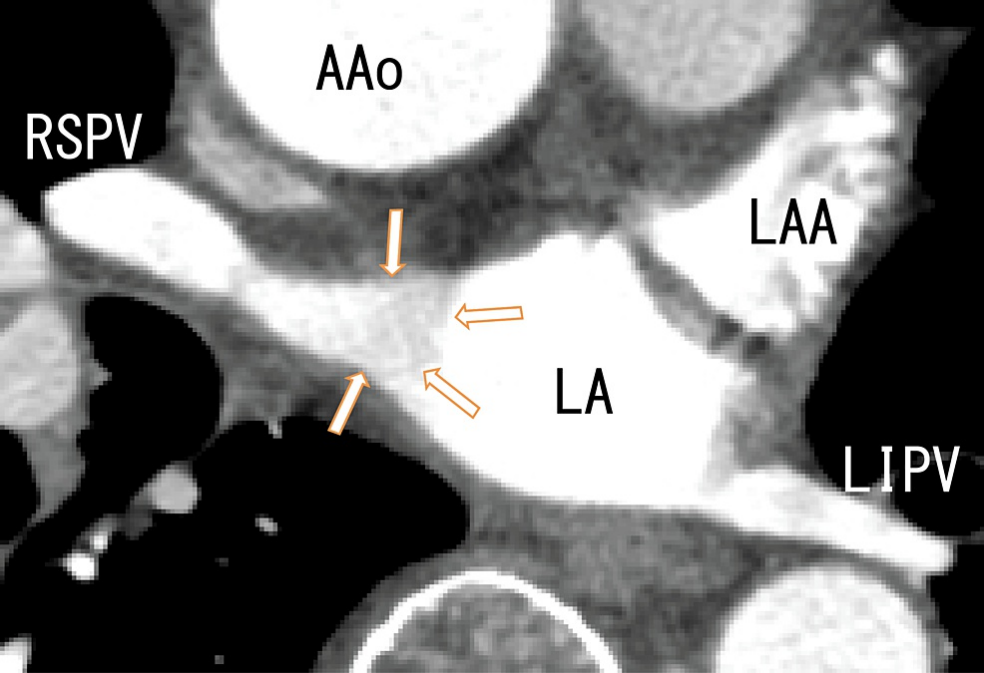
Axial images from an 80-MDCT scan obtained after five months of standard-dose warfarin remedy showing LA thrombi Axial images from an 80-MDCT scan obtained after five months of standard-dose warfarin remedy showing RSPV, LIPV and LA. There were some vague dark images in the LA (arrows), suggesting thrombi in the LA. The dark area of the RSPV became clearer, suggesting that the thrombi had resolved. AAo, ascending aorta; LAA; left atrium appendage; LA, left atrium; LIPV, left inferior pulmonary vein; RSPV, right superior pulmonary vein; 80-MDCT, 80-slice multidetector computed tomography
